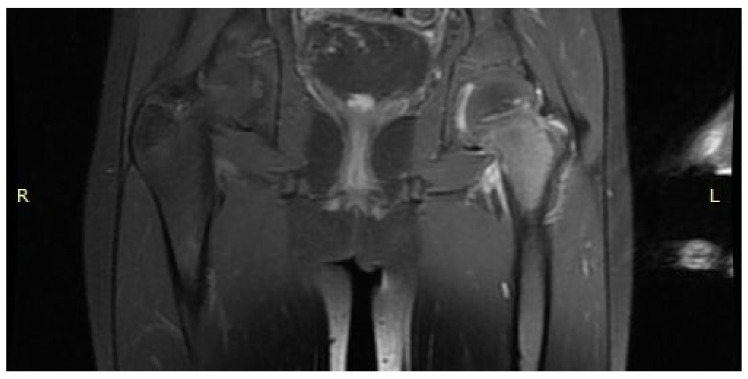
MRI scan of the hip joints showing oedema of the left femoral neck and suggesting inflammation of the hip joint, no nidus revealed.
